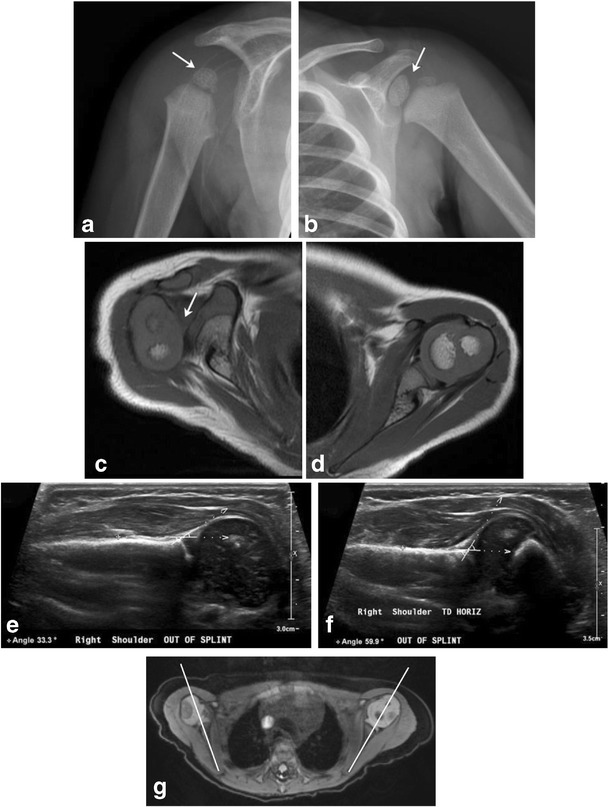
Glenohumeral dysplasia in two neonates Follow-up shoulder imaging in a 13-month-old child with clinically suspected brachial plexus palsy at birth (a-c). He subsequently developed glenohumeral dysplasia. The right humeral head is small, glenoid is shallow as seen on the radiograph (a); compare with the normally formed left humeral head and glenoid labrum (b). Axial T1 MR image (c) reveals posterior subluxation of humeral head; compare to normally aligned left humeral head (d). 2-month-old with history of right brachial plexus injury post-delivery (e-g). Ultrasound images from two exams performed 1 month apart (e and f) demonstrate progressive right glenohumeral dysplasia with interval increase in right humeral alpha angle from 33 degrees to 60 degrees. Axial DESS image from a follow-up MR exam (g) shows a shallow right glenoid with posterior humeral head subluxation. Normal left glenohumeral joint
